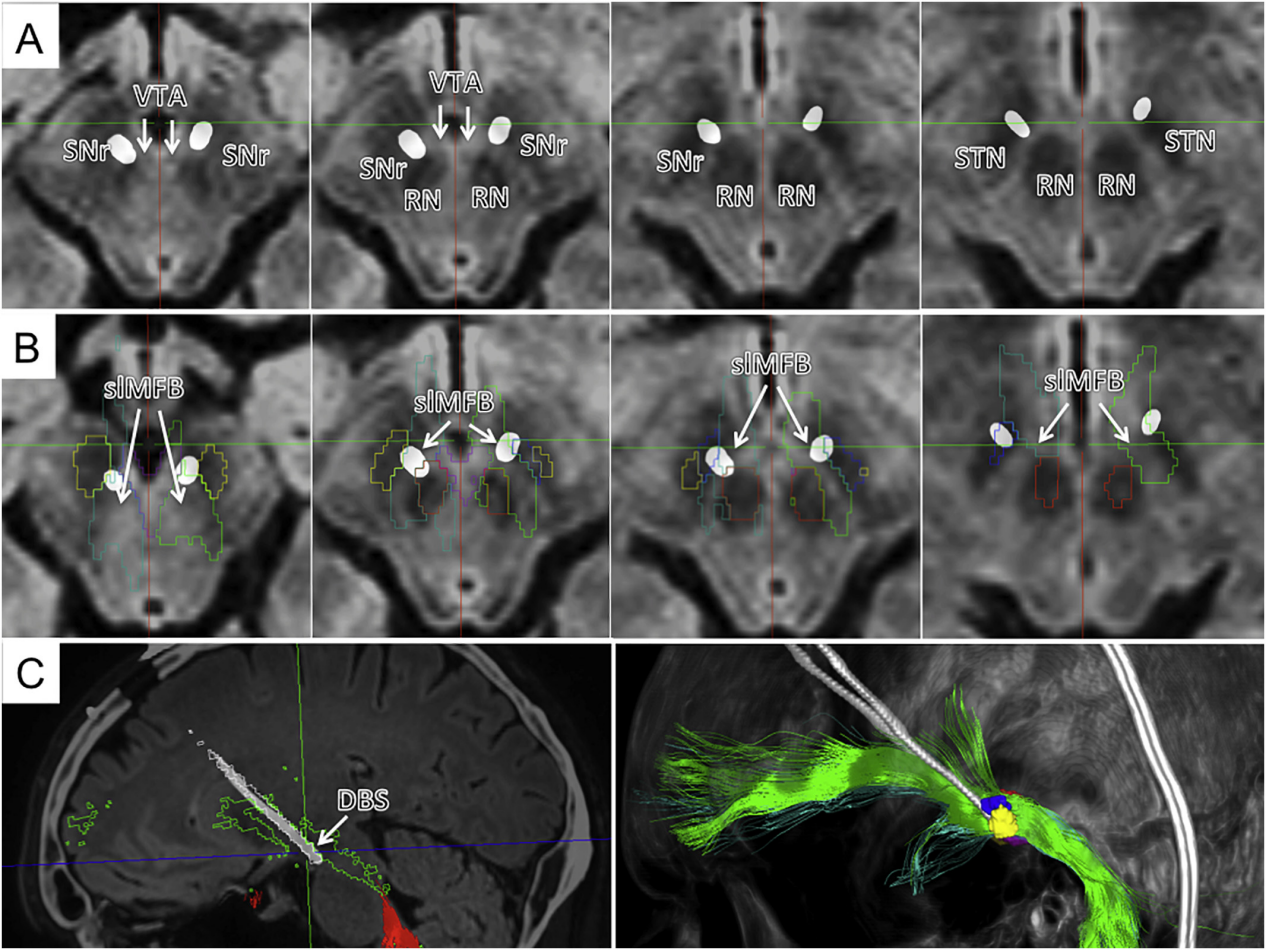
Typical slMFB DBS. A, axial slides showing deepest (left) and most superficial contacts (right) on T2-weighted anatomy. B, Outlines of functional structures given. C; left, outline shows how DBS electrode traverses the slMFB (green); right, three-dimensional view from lateral and left.
